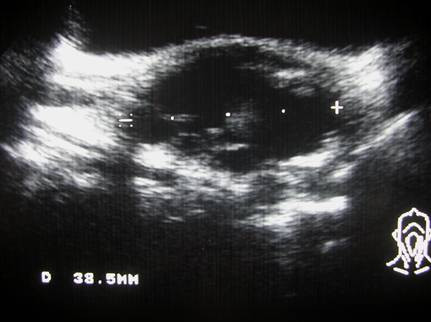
Neck ultrasound evaluation showed a mixed solid and cystic lesion with septations, and irregular and thick margins in lateral region.
